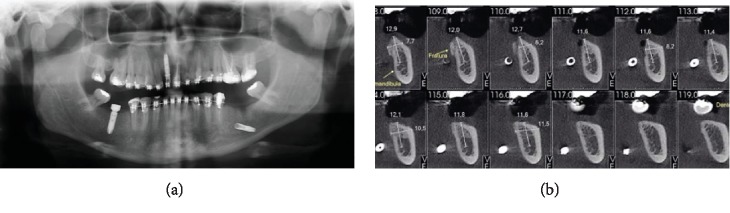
(a) Panoramic radiographic image. (b) Cone beam computed tomographs in sagittal sections showing displacement of the dental implant and fracture of the mandibular lingual cortical bone adjacent to the region of tooth #36.
